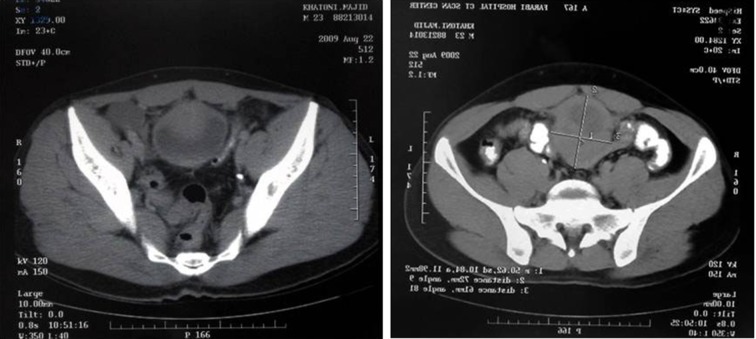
CT Scanning Showed Solid Mass Above the Bladder
